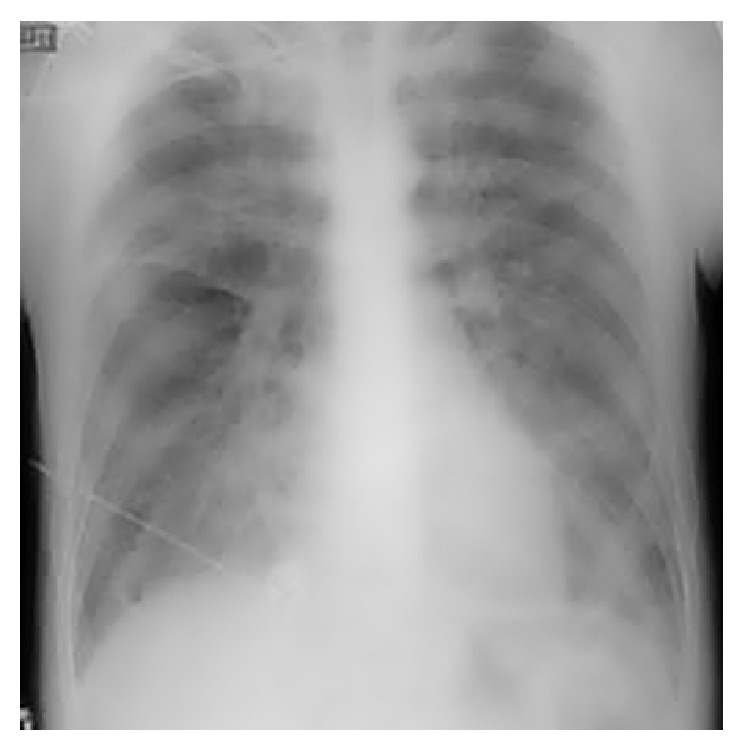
Chest radiograph showing pulmonary edema with normal cardiac area.
